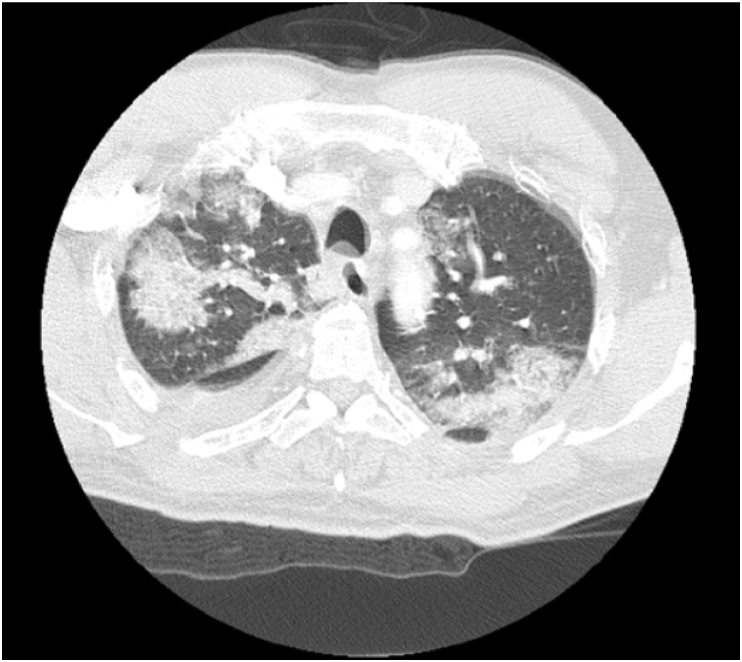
Axial CT chest on day of admission with multifocal mixed ground glass and dense consolidations throughout bilateral lungs.
